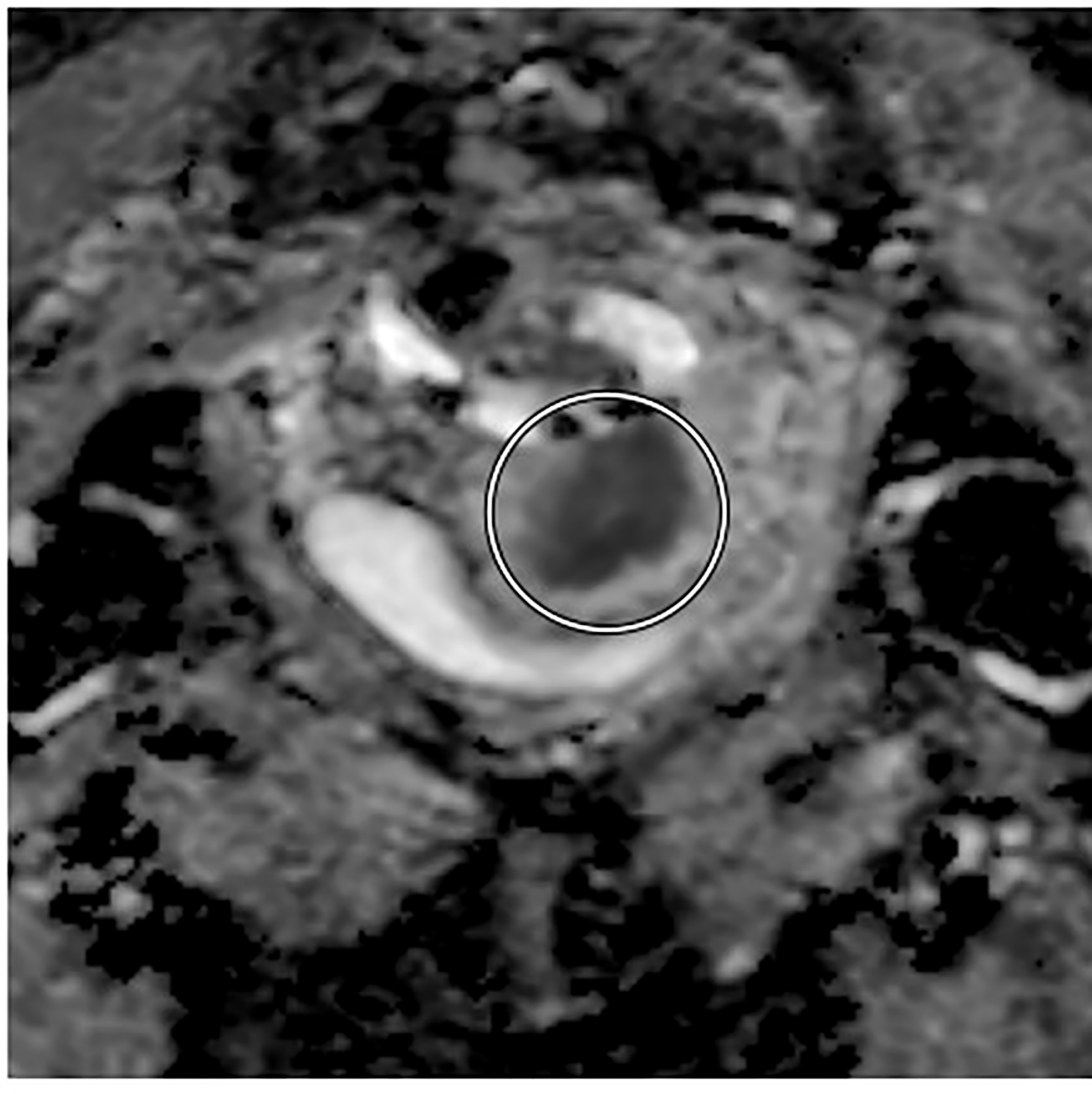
Axial diffusion-weighted MRI showing pre-treatment decreased tumor signal intensity in a patient with FIGO IIIC1, pathologic T2 squamous carcinoma of the cervix.
